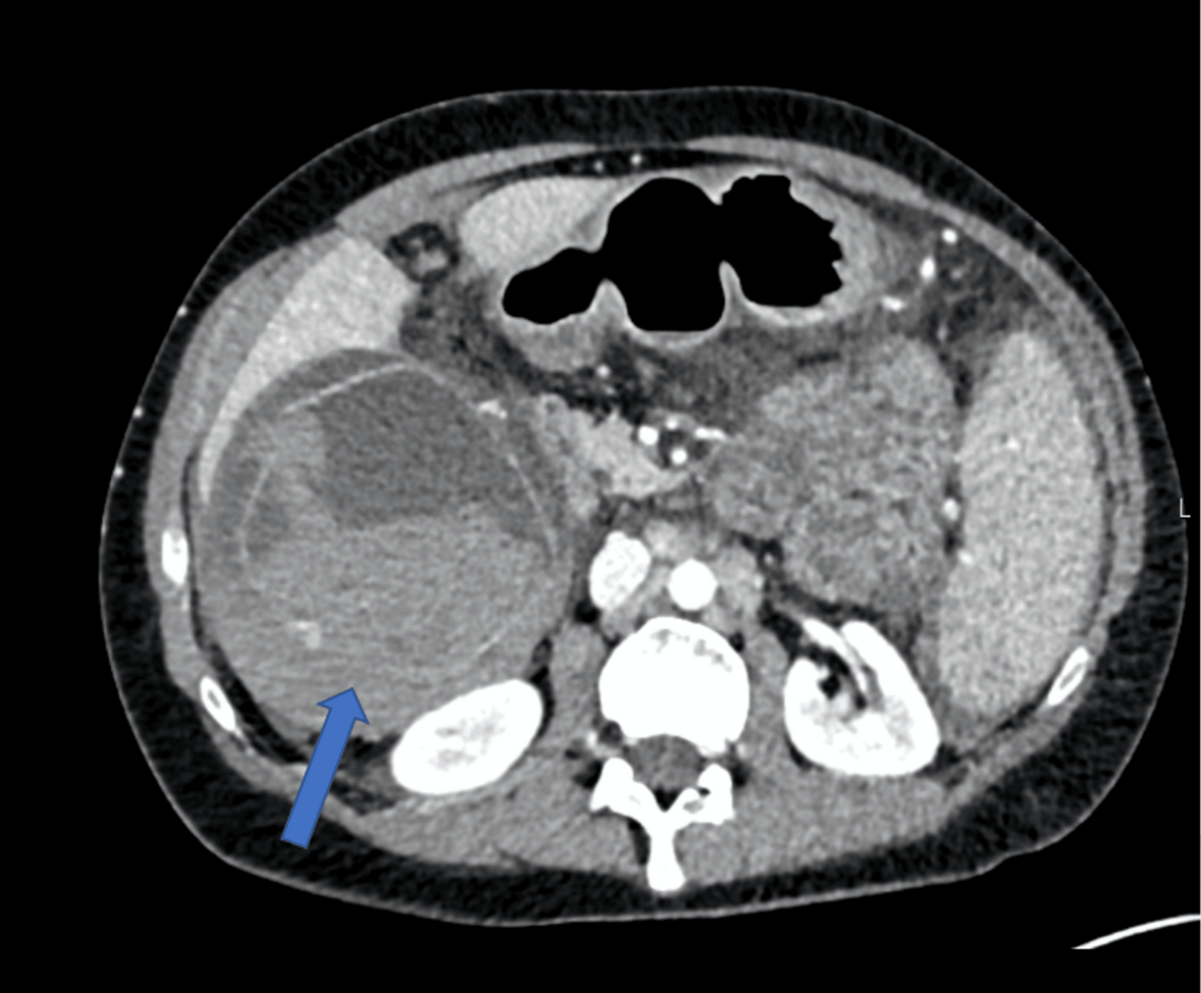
CT imaging of a case of haemorrhagic gallbladder. The gallbladder appears distended, containing a large amount of blood. A defect in the posterior gallbladder wall is noted, suggesting the site of rupture (arrow).
